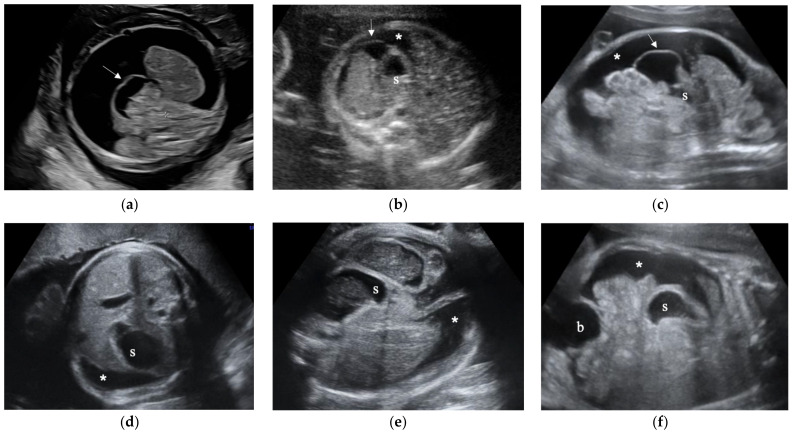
Ultrasound images of fetal ascites. (a) case 4, 22 weeks of gestation, (b) case 6, 26 weeks of gestation, (c) case 7, 28 weeks of gestation, (d) case 9, 31 weeks of gestation, (e) case 10, 39 weeks of gestation. (f) case 11, 28 weeks of gestation. Asterisk (*): ascites, arrow: greater omentum, s: stomach, b: bladder.
